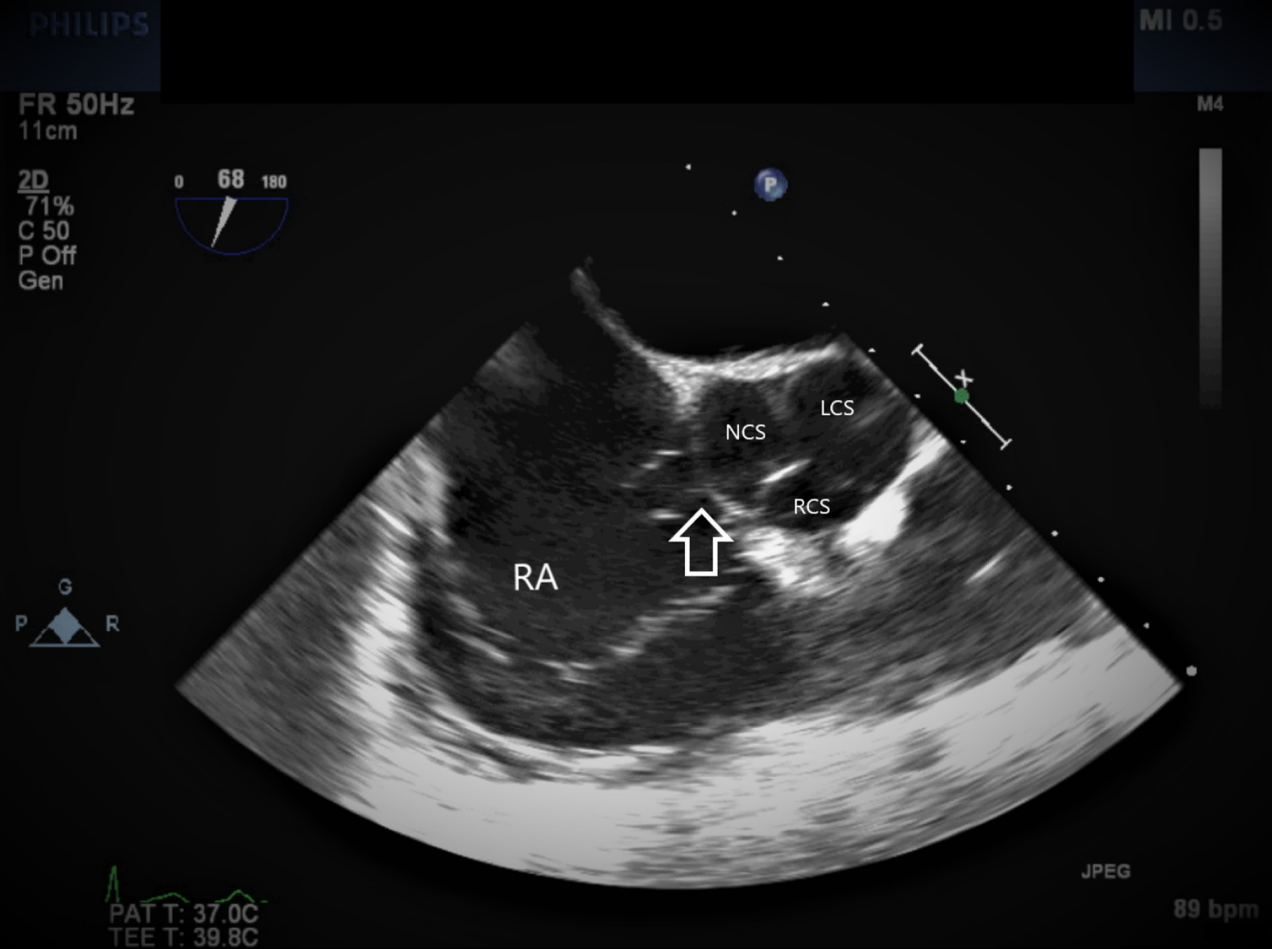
TEE image at mid-esophageal aortic valve short axis view The arrow showing SVA arising from the noncoronary sinus. TEE, transesophageal echocardiogram; SVA, sinus of Valsalva aneurysm; RA, right atrium; NCS, noncoronary sinus; LCS, left coronary sinus; RCS, right coronary sinus.
